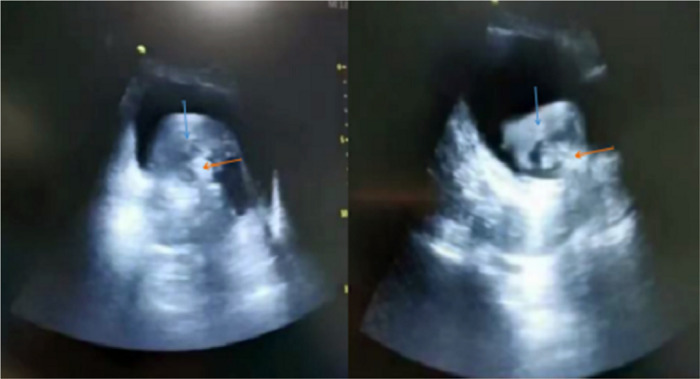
The morcellator in the centre of the blood clots under ultrasound guidance (blue arrow: blood clot; Red arrow: morcellator).
